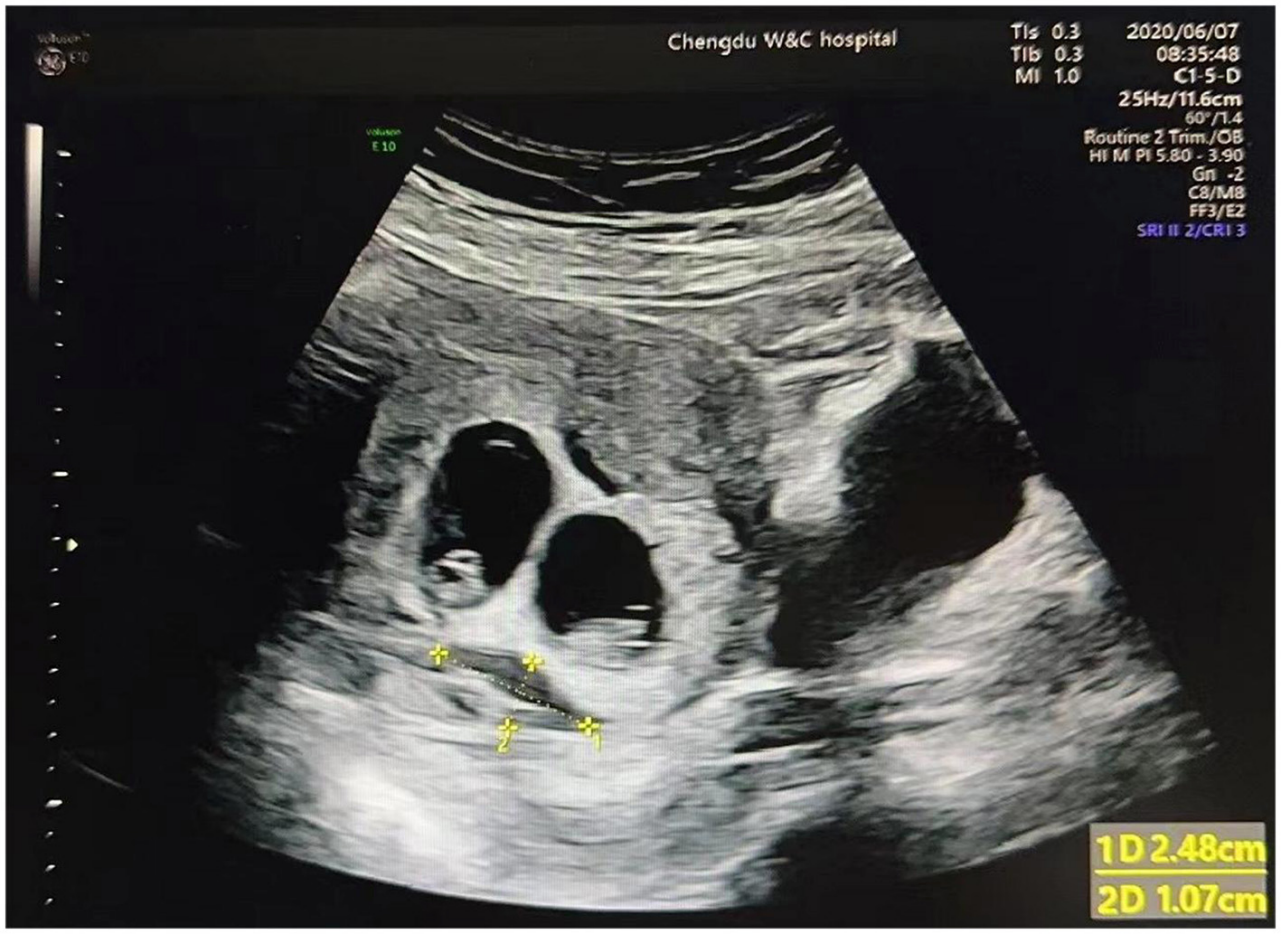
Ultrasound image for SCH in twin pregnancies.
